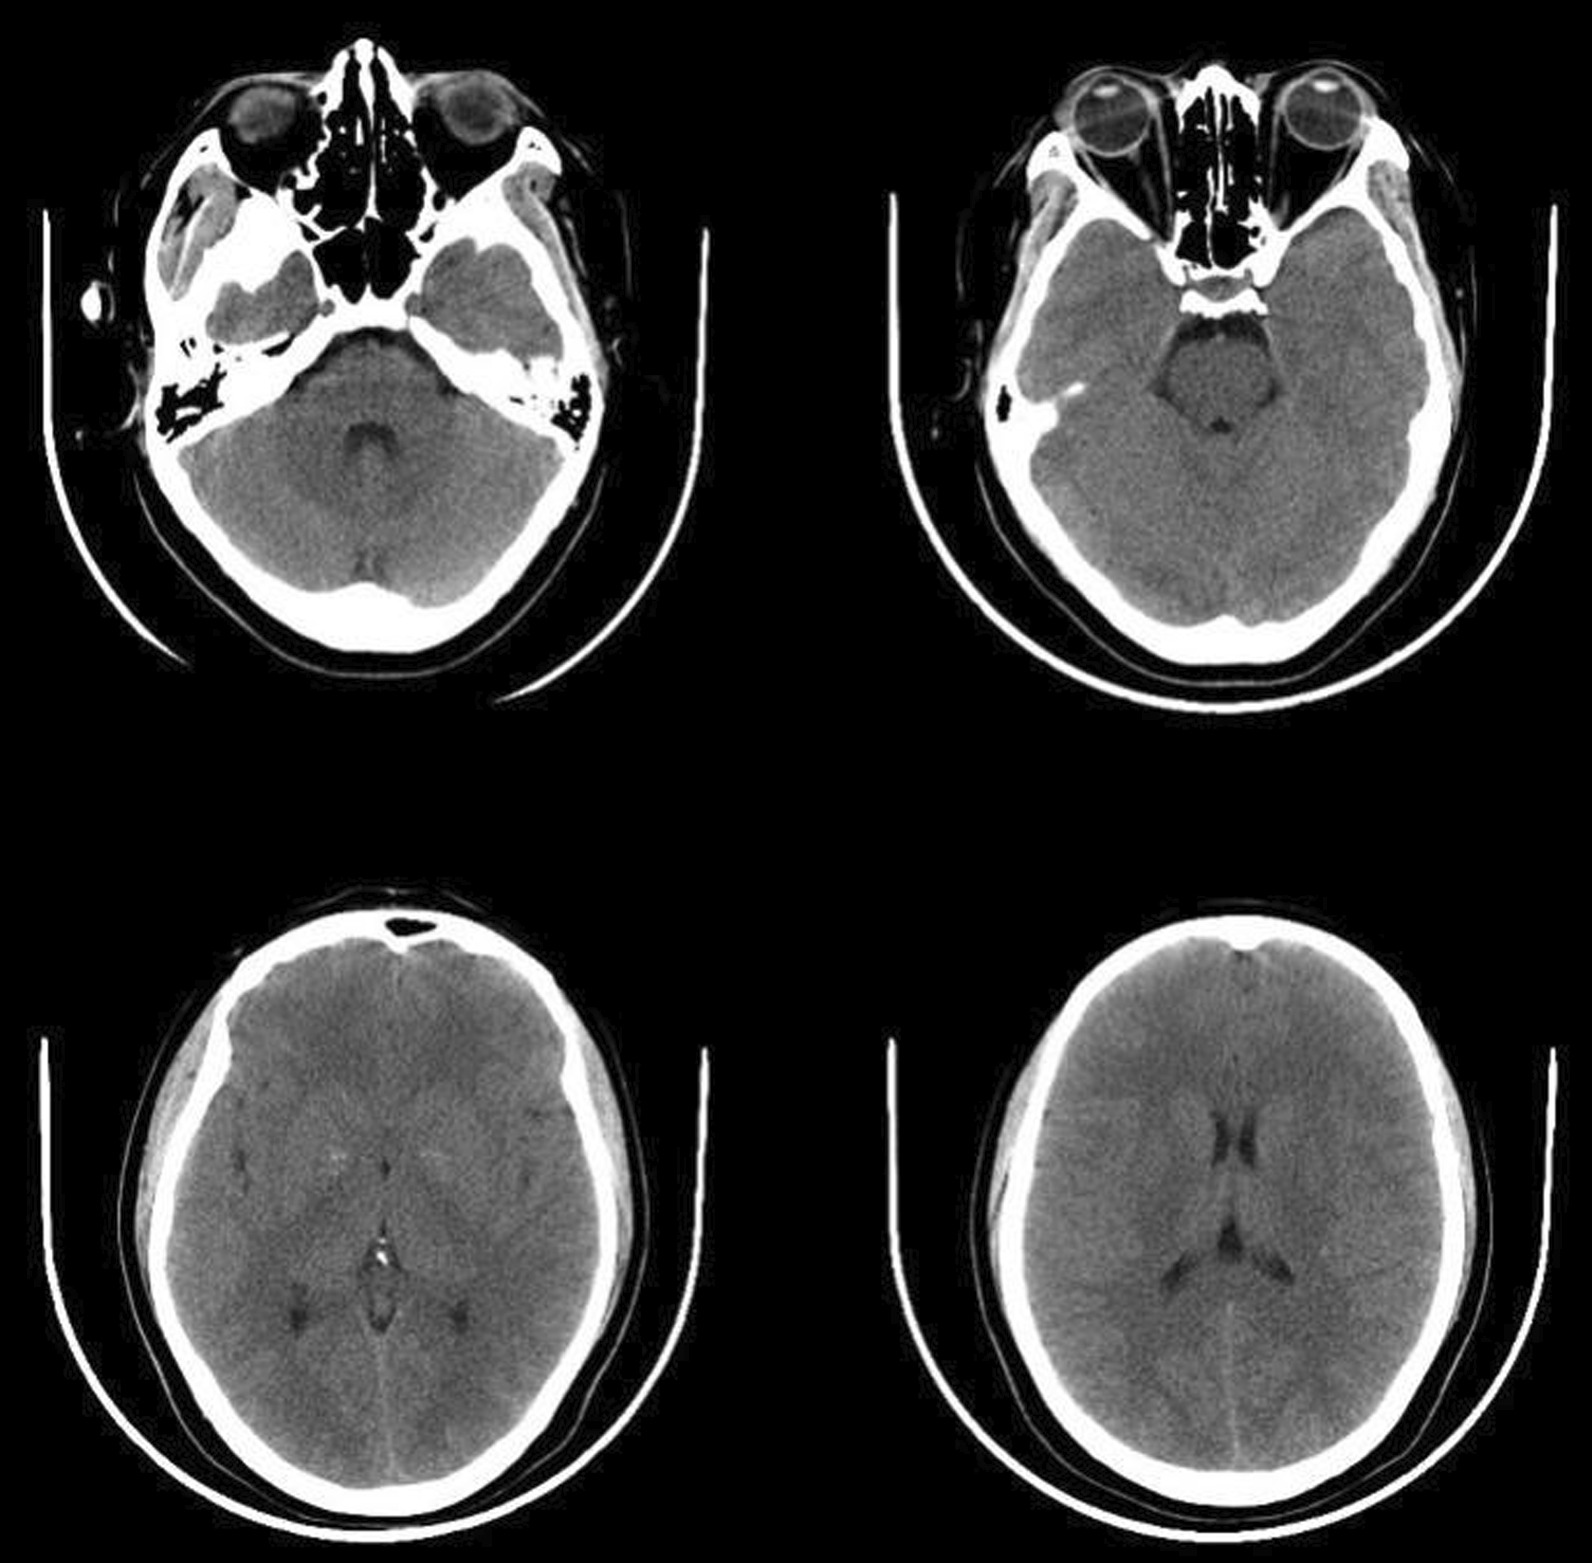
Swelling of brain parenchyma (cranial computed tomography)
